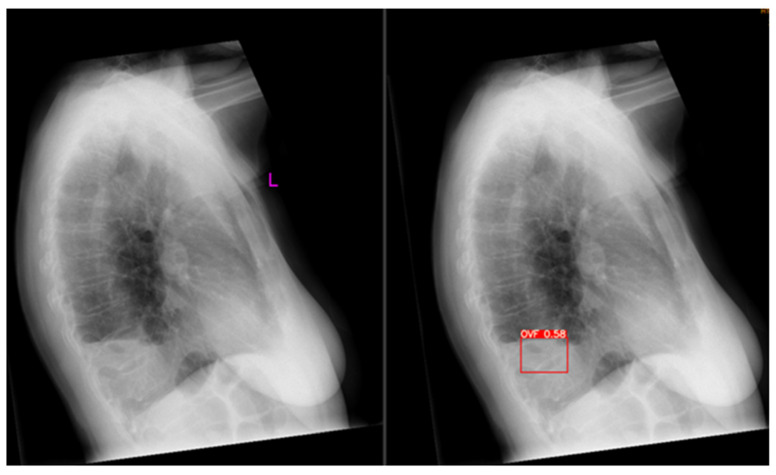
Example of a false positive. The original radiograph is depicted on the left; Ofeye 1.0 analysis with flagged OVF is indicated in red on the right. The radiologist did not classify this as an OVF. The total false positive rate was 5.1%. L, left.
